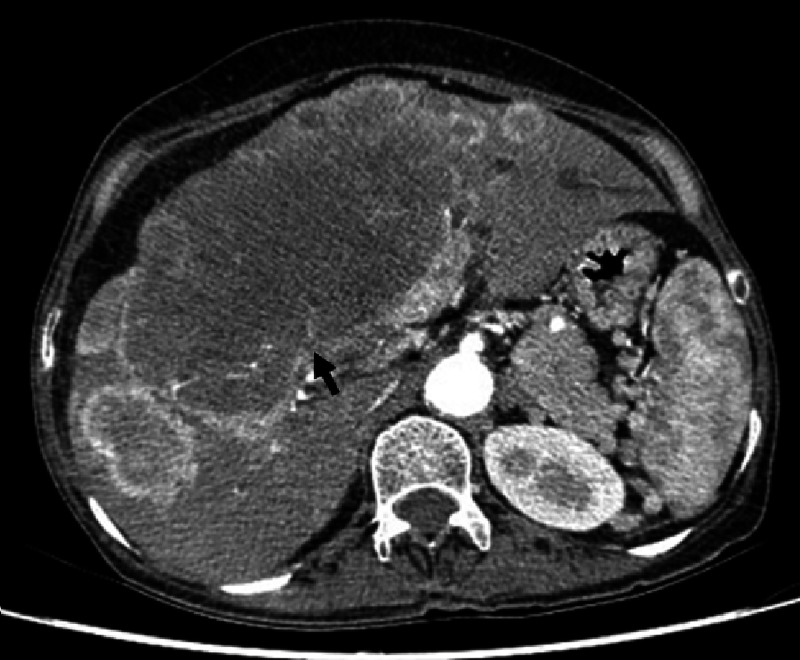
Liver MRI. Infiltrative lesions in segments IV, V, III, VII, and VIII.
